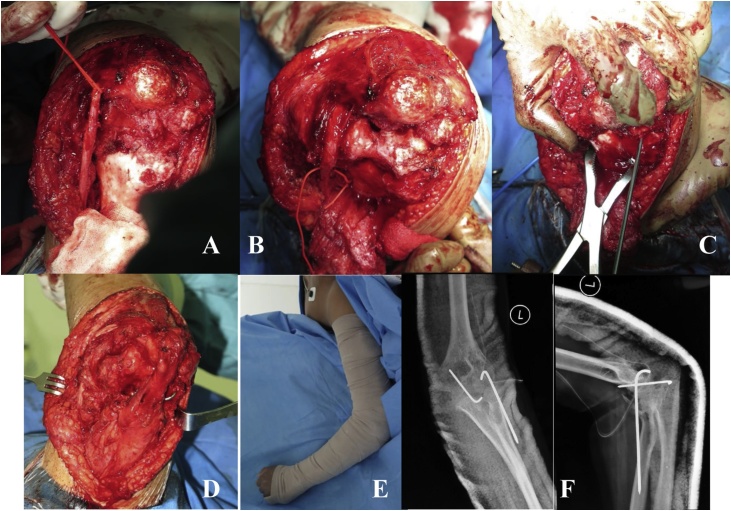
Ulnar nerve and heterotopic ossification identification (A), contracture release and ulnar nerve preservation (B), open reduction and internal fixation using K-Wire (C), final exposed and ulnar nerve transposition (D), immobilization using backslab in 90^0^ flexion position (E), post-operative X-Ray (F).
